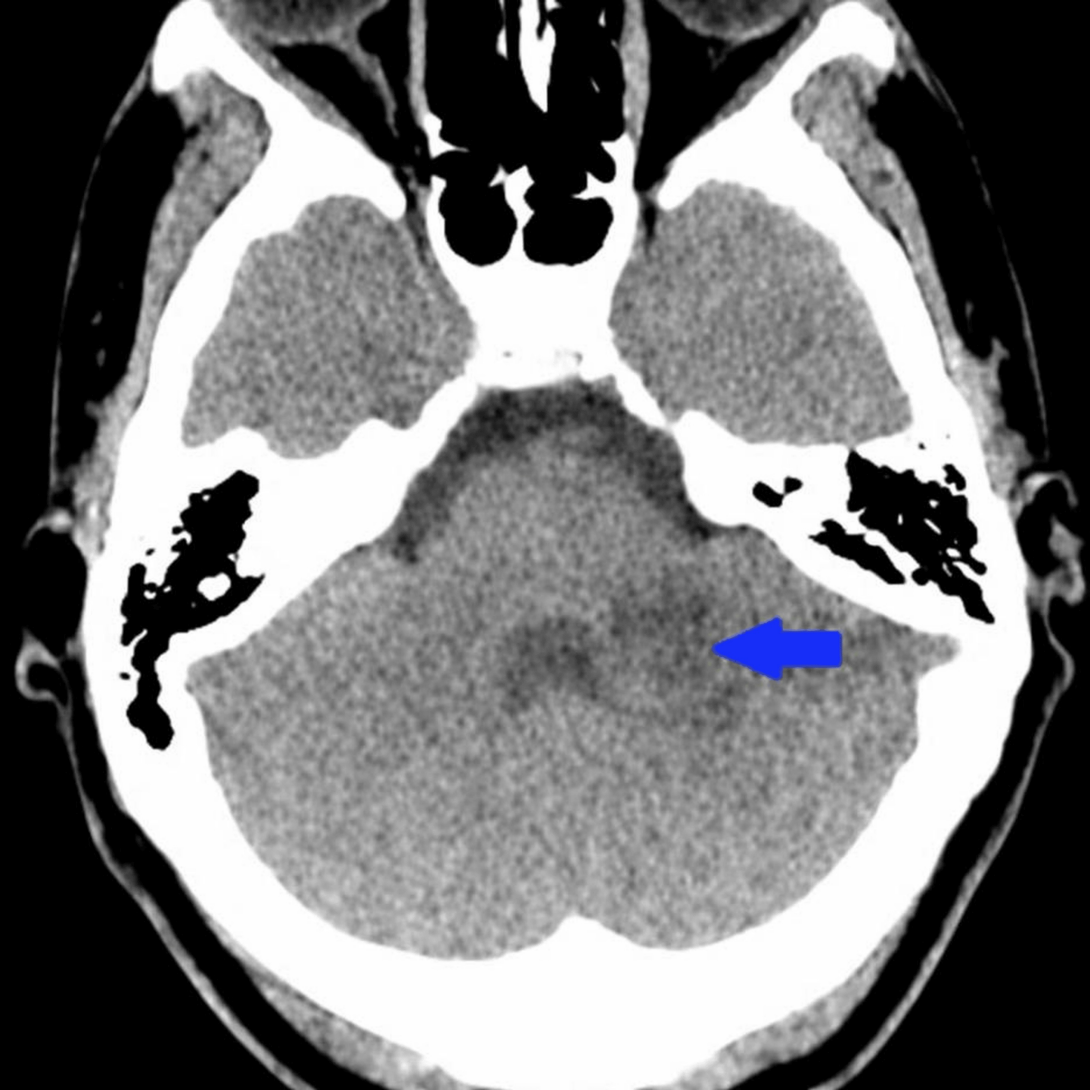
Non-contrast axial CT of the brain obtained in October 2025, performed nine hours after return of spontaneous circulation, demonstrates an approximately 3-cm hypodense lesion in the left cerebellar hemisphere (arrow), consistent with acute to subacute infarction, without hydrocephalus or significant mass effect.
